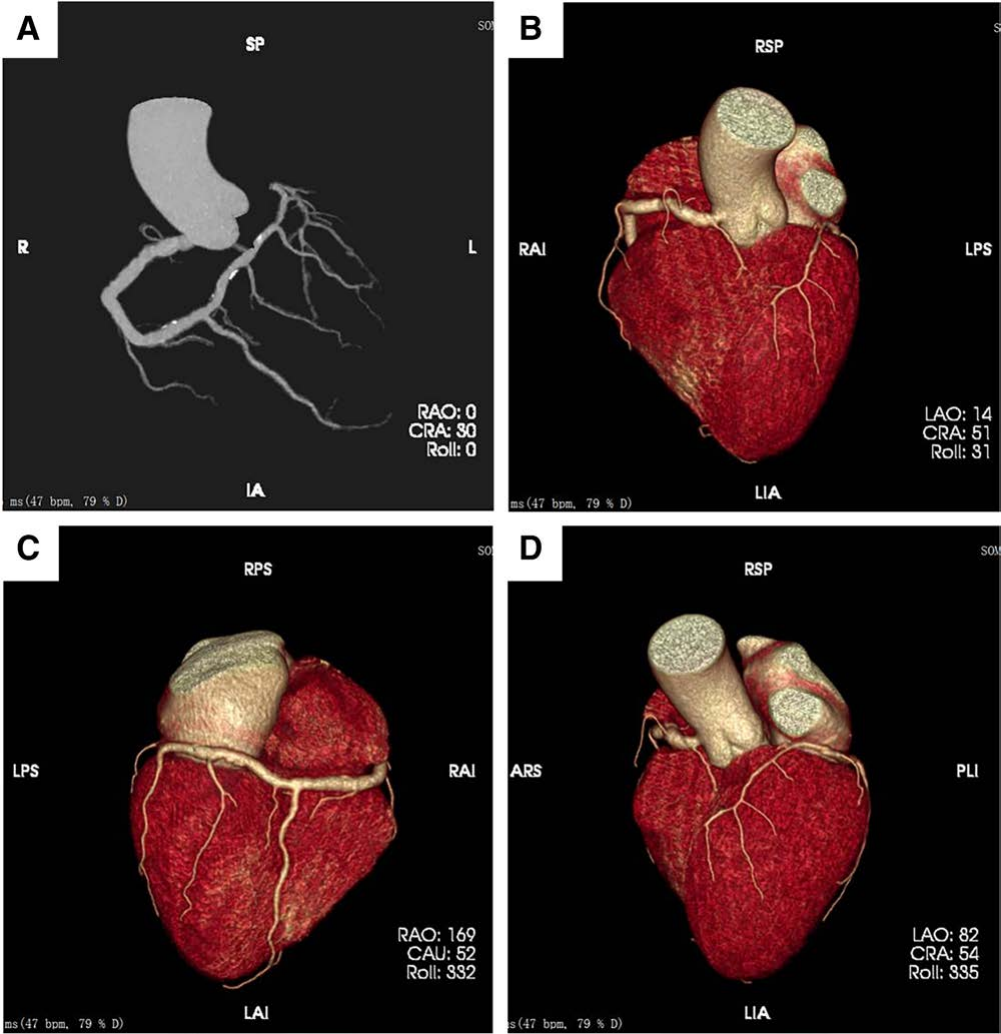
The images of CTA at 6 months after discharge. (A) A single RCA arising from the right coronary sinus with branches supplying the left coronary territory, with patent lumen and no severe stenosis. (B–D) Three-dimensional reconstruction of the anatomical course of the RCA. The RCA emanated from the right sinus and travels in the right atrioventricular groove to the posterior crucifixion where it divided into a posterior descending branch that traveled to the apex and bypasses the apex to supply the posterior interventricular septum and the apical region of the heart, and gave off a diagonal branch that supplied blood to the left ventricular side wall and anterior wall. CTA = computed tomography angiography, RCA = right coronary artery.
